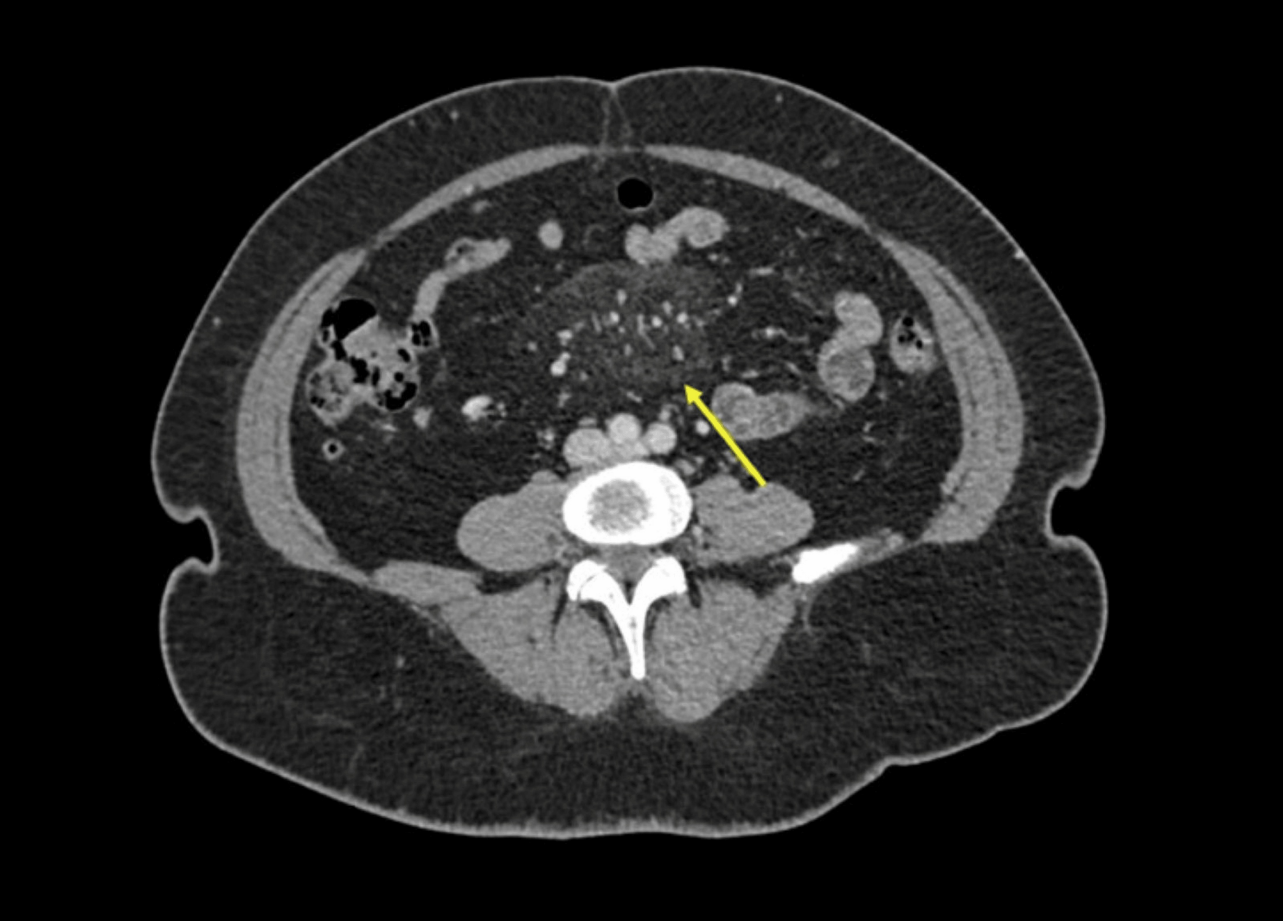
Contrast-enhanced abdominal CT scan showing increased density of mesenteric fat in the mesogastrium with some reactive lymph nodes (yellow arrow) due to mesenteric panniculitis.
